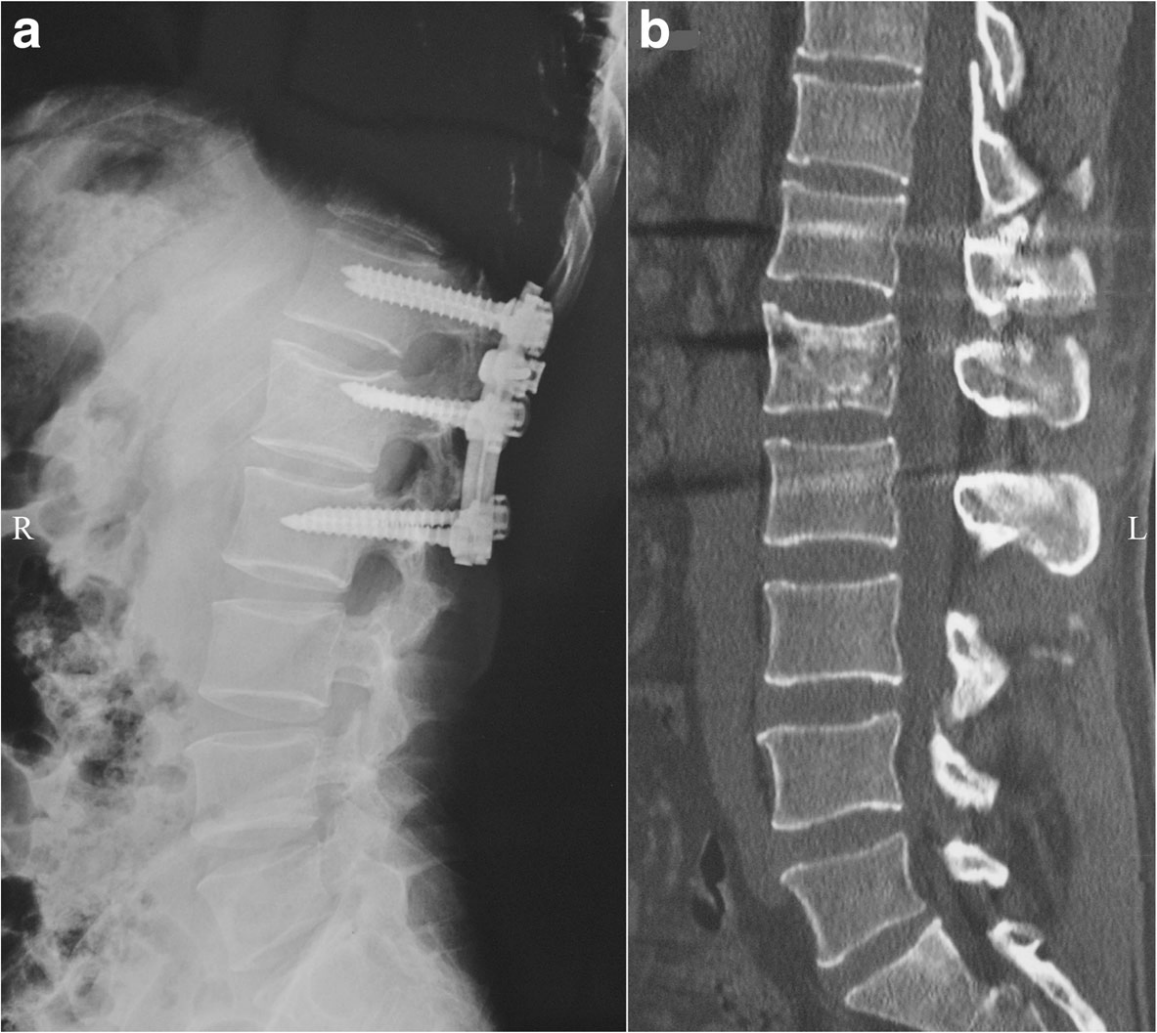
a Lateral radiograph of the patient shown in Fig. 2 at 1 year postoperatively. b CT of the patient shown in Fig. 2 at 1 year postoperatively
